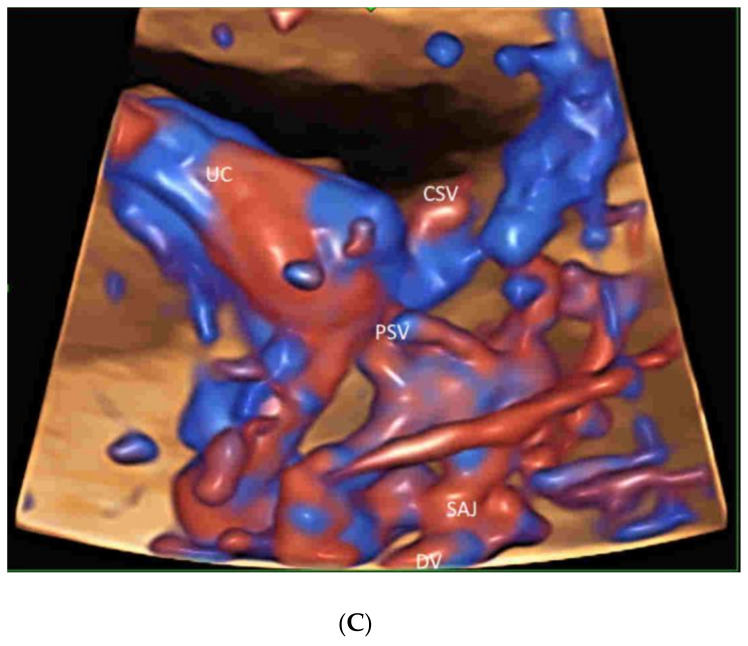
Microvascular flow imaging of placental (P) vessels in two normal pregnancies at 13 weeks’ (A) and at 23 weeks’ (B) gestation. Note the demonstration of the spiral artery jet (SAJ) and the primary (PSV) and secondary (SSV) stem villi in (A). In addition, the tertiary stem villi are demonstrated in (B). Three-dimensional ultrasound in high-definition flow displayed in the glass-body mode showing the spatial relationship of the placental vessels at 20 weeks’ gestation (C). Umbilical cord, UC; DV, decidual vessels, CSV, chorionic surface vessels; AF, amniotic fluid.
